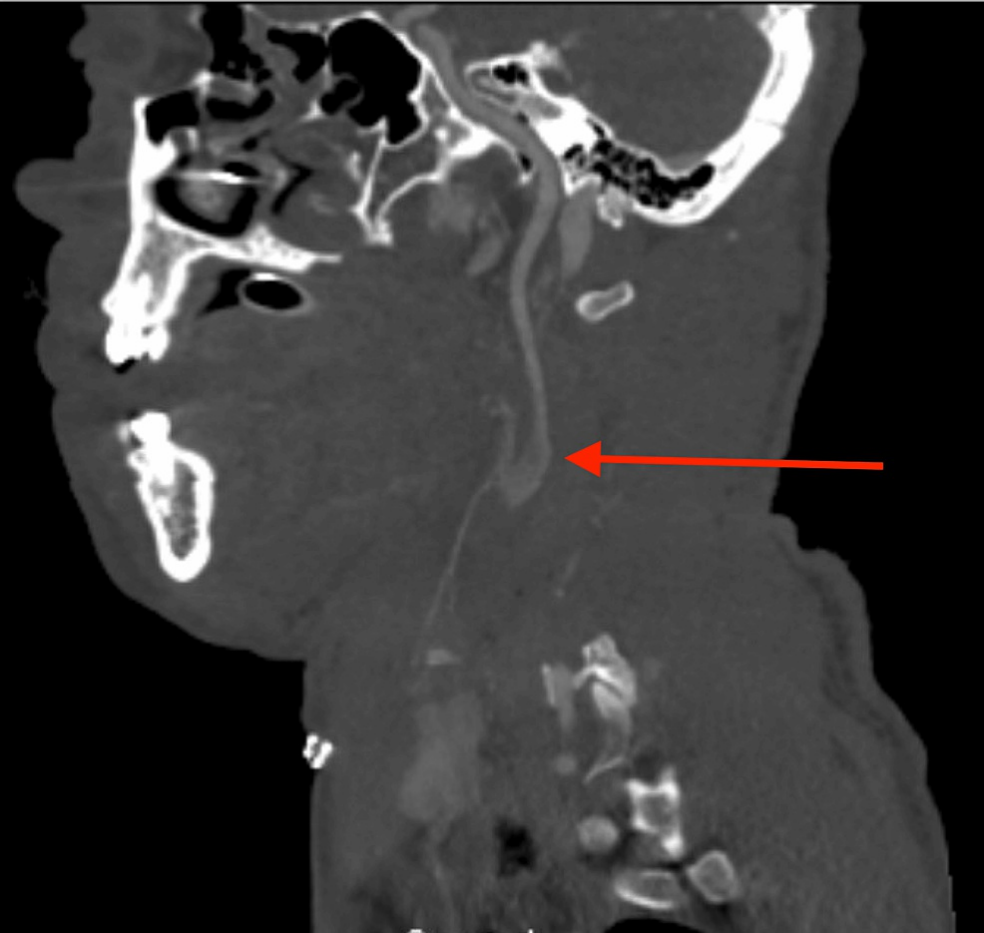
CT angiography of the neck in sagittal views, demonstrating backflow through the external carotid artery up into the internal carotid artery
